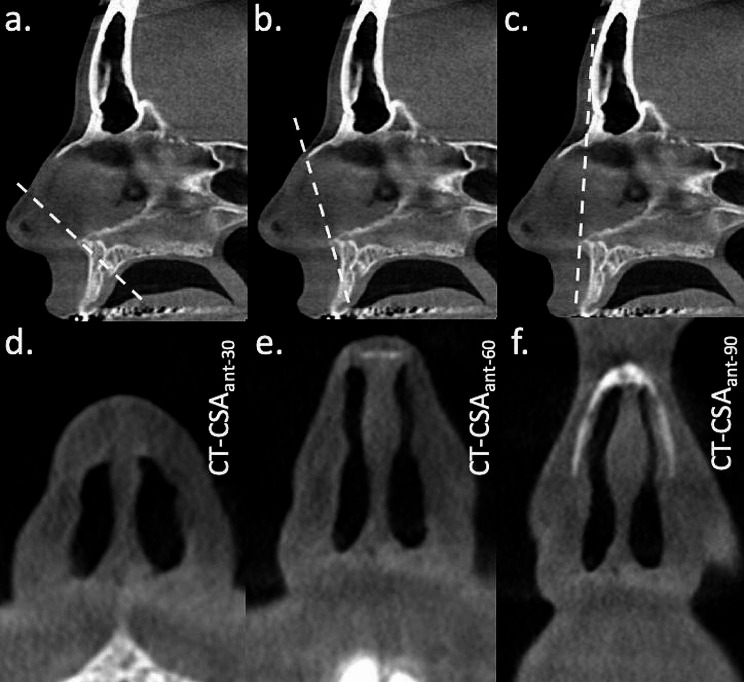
Identification of three planes approximately perpendicular to the curved airflow in the anterior nose in a mid-sagittal section. The white dashed line indicates the selected oblique planes using the anterior nasal spine as a pivot point to the (a) posterior edge of the inferior ostium of the incisive canal, (b) anterior edge of the intranasal suture and (c) most ventral part of the frontal bone. The corresponding cross-sectional areas are shown below: (d) CT-CSA_ant−30_, (e) CT-CSA_ant−60_, and (f) CT-CSA_ant−90_. Note the absence of mucosal structures
